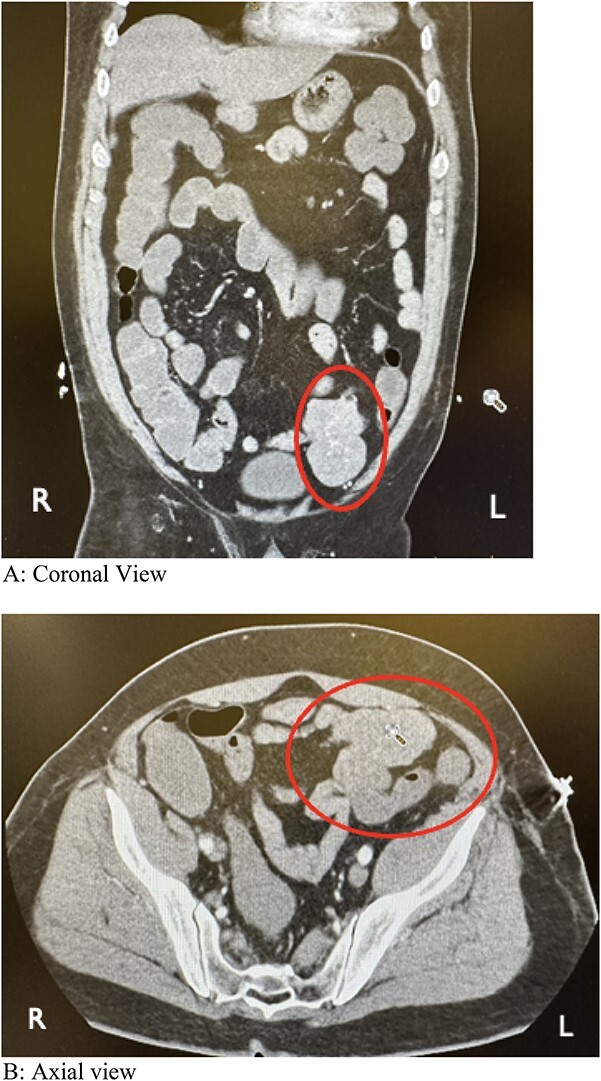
Computed tomography angiography showing a lobulated soft tissue mass associated with distal small bowel loops in the left lower quadrant. (A) Coronal view. (B) Axial view.
